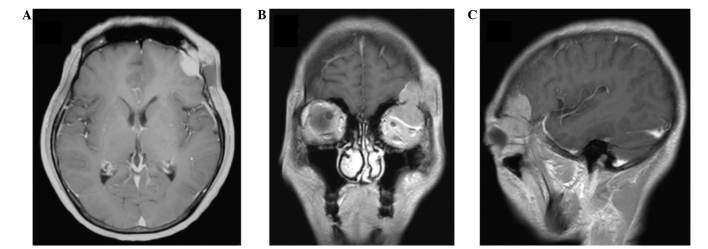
T1-enhanced magnetic resonance imaging of the patient revealed (A) dural attachment and a mild dural tail sign, and (B) coronal and (C) saggital plane images revealed a cranio-orbital invasive lesion with moderate enhancement.
